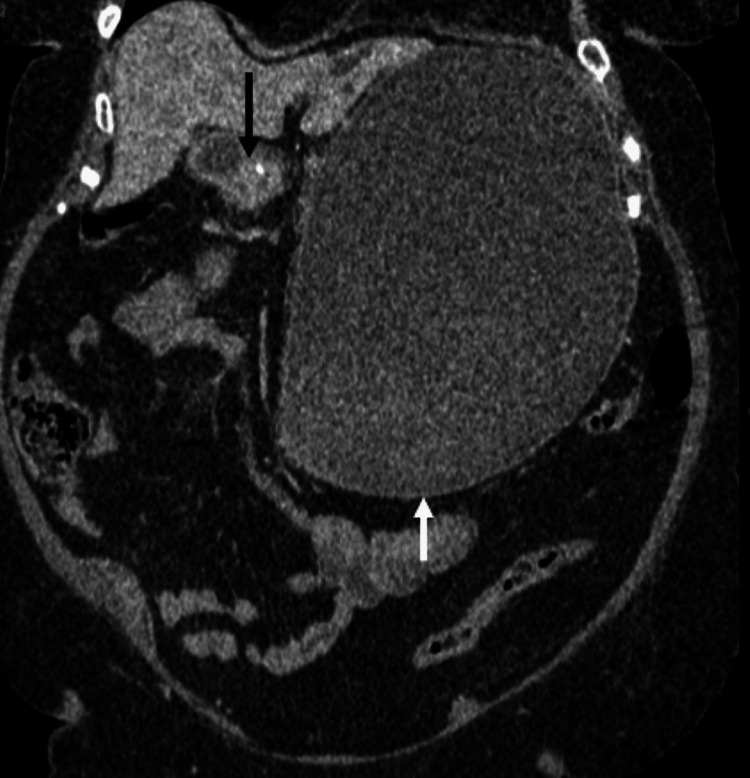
Coronal portal venous CT, as previous image, showing gallbladder adherent to duodenum with a calcified opacity representing a gallstone within the duodenal lumen (black arrow). Also note massive gastric distension (white arrow).
